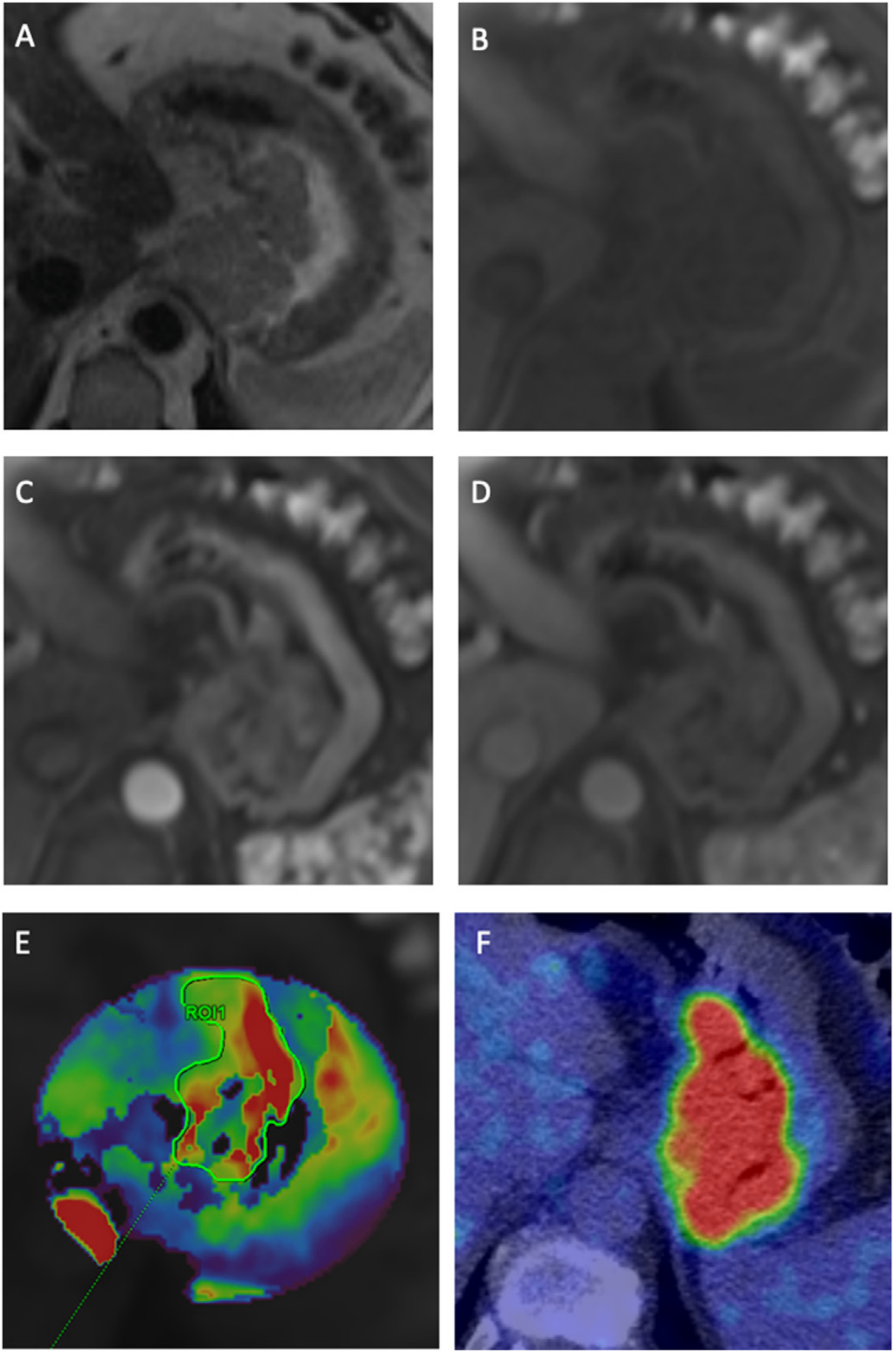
59-year-old with an esophagogastric adenocarcinoma responding to neoadjuvant therapy (TRG 2). Axial T2-weighted MRI (A) demonstrates the primary tumor. Axial pre-contrast T1-weighted MRI (B), arterial phase T1-weighted MRI (C) and portal-venous phase T1-weighted MRI (D) show early tumor enhancement with washout. Corresponding peak enhancement map (E) and ^18^F-FDG PET/CT (F) show lower tumor peak enhancement of 0.17 mmol.L^−1^ and metabolic activity, SUV_max_ of 29.8, respectively.
